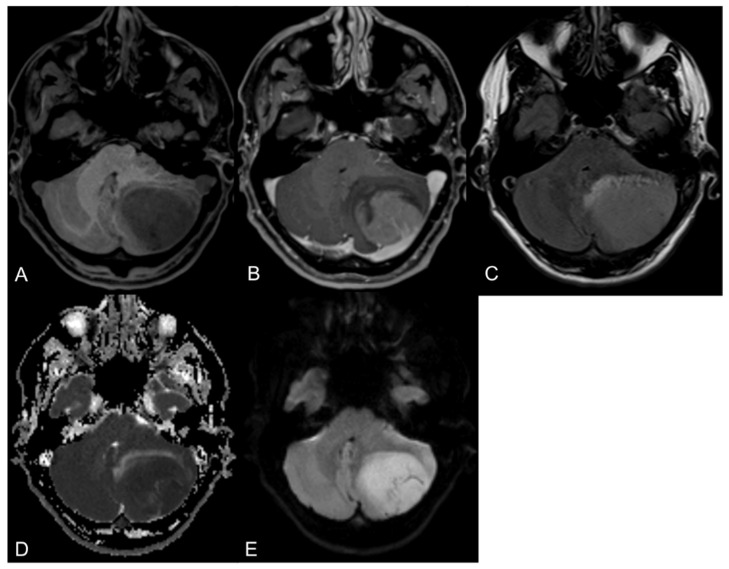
Axial MR images of a 46-year-old male patient with a medulloblastoma located in the left cerebellar hemisphere. The tumor shows a characteristic hypointense signal on T1-weighted images (A) with a mainly homogenous contrast enhancement (B), a hyperintense signal on FLAIR images (C) and a considerable diffusion restriction (D,E).
